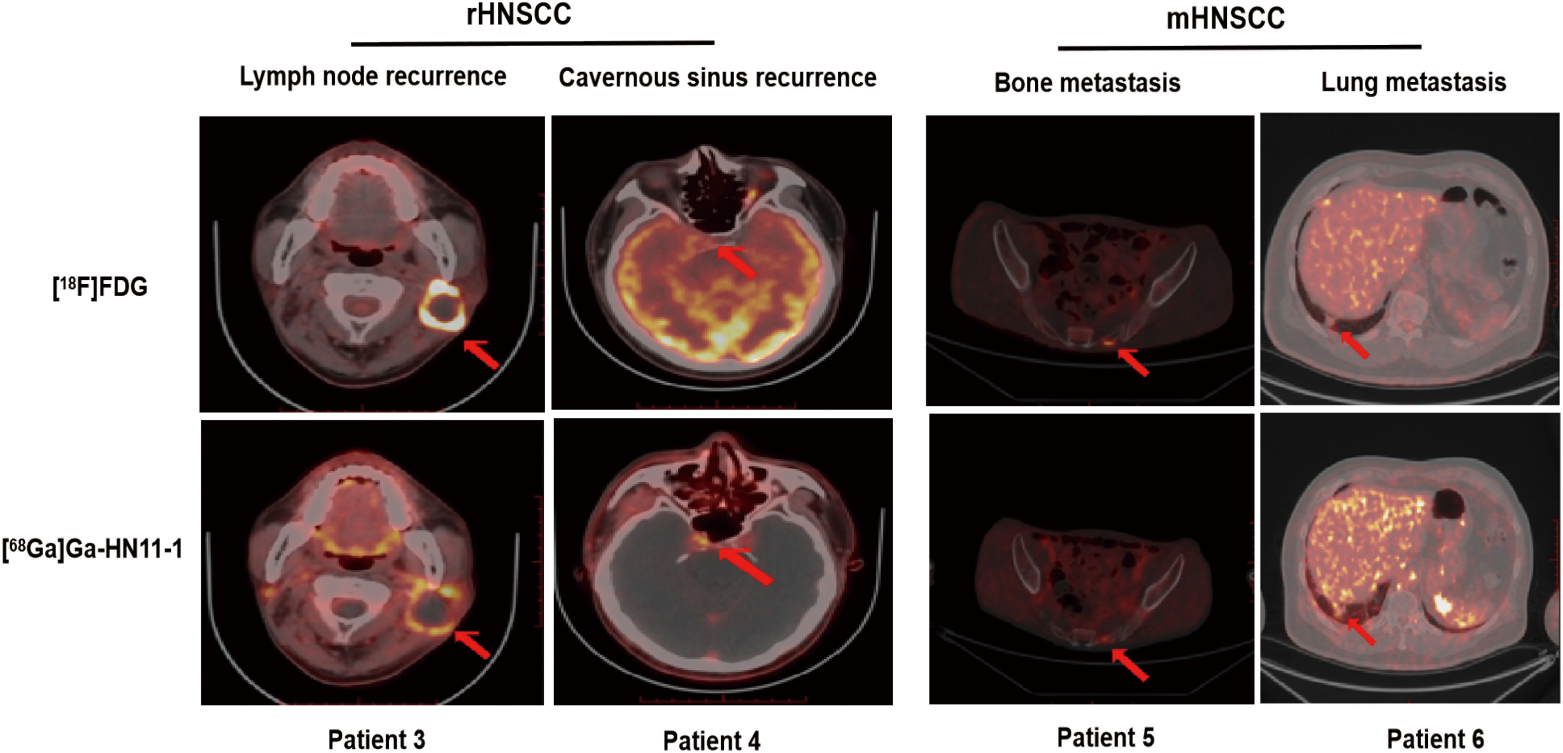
[^68^Ga]Ga-HN11-1 and [^18^F]F-FDG PET/CT imaging in recurrent and metastatic HNSCC patients (tumor location is indicated by the red arrow). Patient 3 and patient 4 were transverse imaging for recurrent HNSCC. Patient 5 and patient 6 were transverse imaging for bone and lung metastases of HNSCC.
